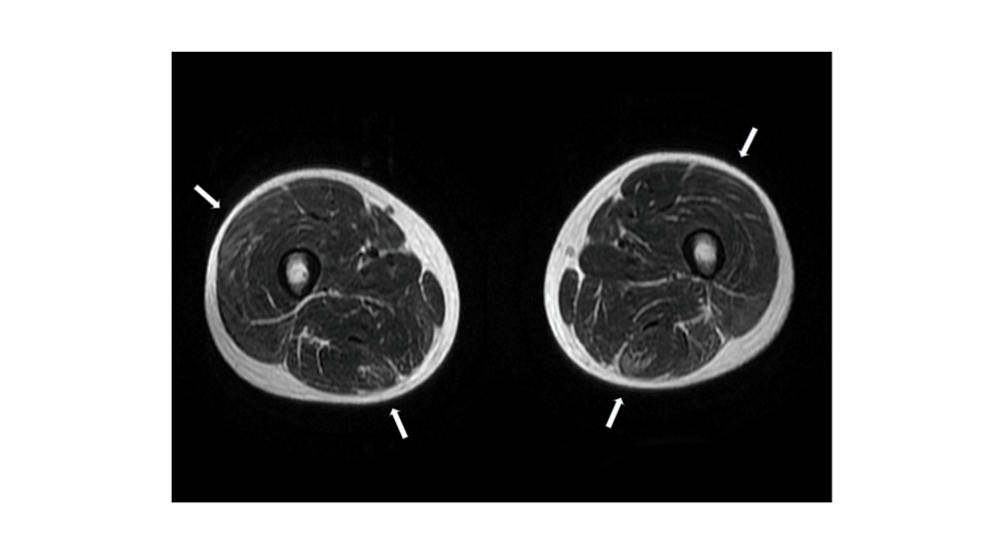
Magnetic resonance imaging of both thighs. Axial T2-weighted image reveals bilateral patchy hyperintense lesions in both muscles.
